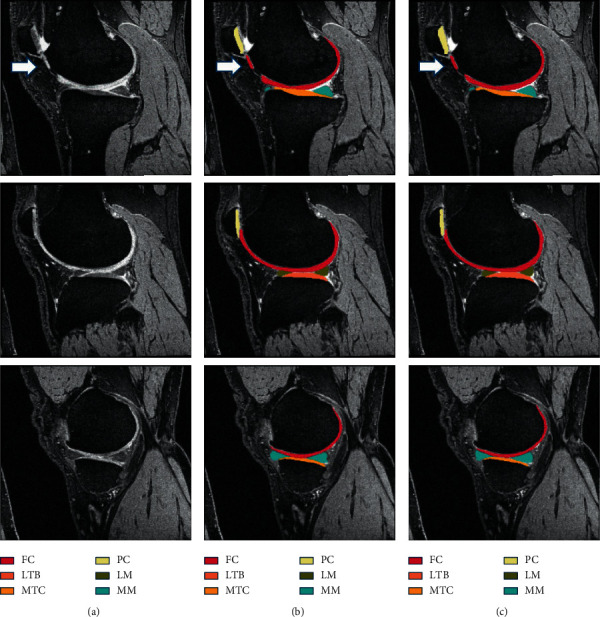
Examples of segmented MR images with different colour codes for different compartments (femoral cartilage (FC), lateral tibial cartilage (LTB), medial tibial cartilage (MTC), patellar cartilage (PC), lateral menisci (LM), and medial menisci (MM)) (adapted from [49]). (a) Original image, (b) manual segmentation, and (c) automatic segmentation.
